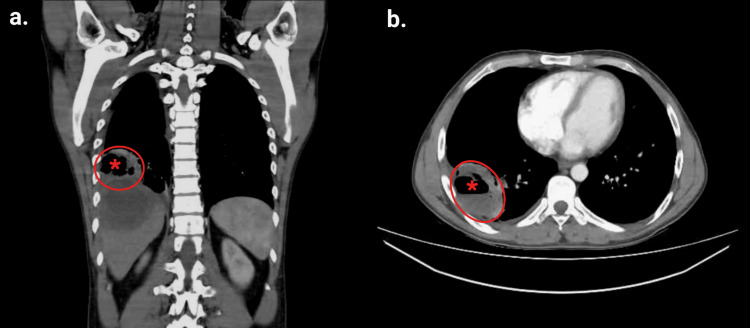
Contrast-enhanced chest CT: (a) coronal view and (b) axial view. A hypodense collection consistent with a pulmonary abscess (red circles) is observed in the anterior and lateral basal segments of the right lower lobe, with an internal air-fluid level (red asterisks) and irregular margins, suggestive of direct extension from the hepatic abscess with secondary infection. The lesion measures 5.1 × 7.8 × 7.3 cm in the anteroposterior, transverse, and craniocaudal dimensions, respectively, and is associated with pleural effusion and adjacent ground-glass opacity. CT: computed tomography
